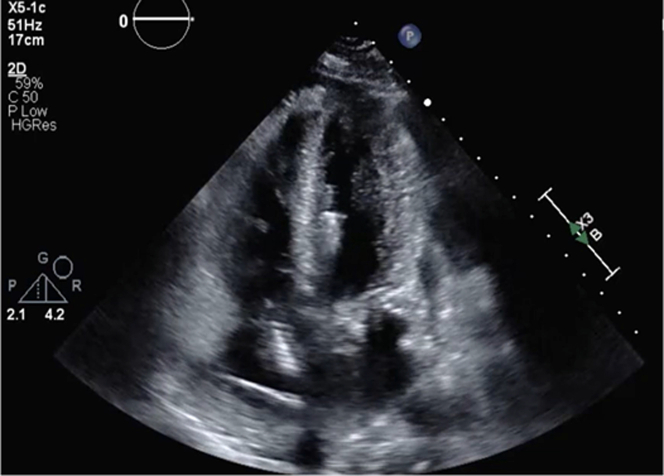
Initial Transthoracic Echocardiogram Findings included an ejection fraction of 45%, moderate concentric left ventricular hypertrophy, and hypokinetic apical inferior septum/anterior segments.
